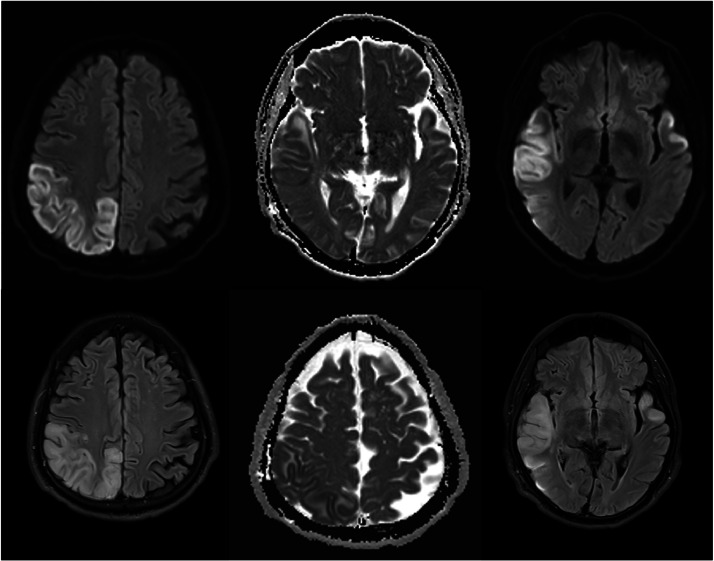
MRI brain (Left to right: DWI, ADC, and FLAIR sequences) demonstrates multifocal areas of cortical based restricted diffusion and FLAIR hyperintensity involving the right MCA/posterior PCA/ACA territories and left anterior temporal lobe.
